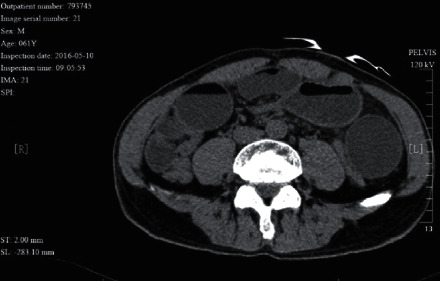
A 61 year-old male patient with adhesive intestinal obstruction complicated with small intestinal volvulus. He had received surgery for rectal cancer 2 years ago. The CT image shows a beak sign in front of abdominal aorta. No bowel wall ischemia was identified during surgery.
